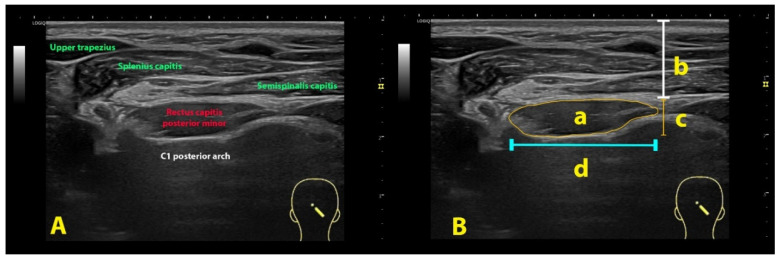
Ultrasound imaging of the rectus capitis posterior minor (A) and its imaging management (B): cross-sectional area (a), depth (b), thickness (c) and width (d) using the rectus capitis posterior minor muscle as example.
